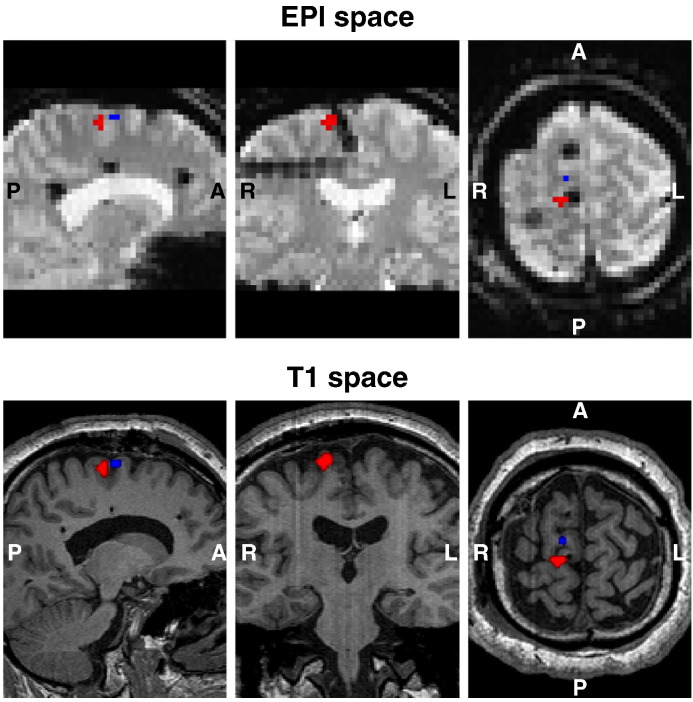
Representative example (patient ID: B, events of interest set: *S*_*2*_, see Table 1) of IED onset and width related BOLD changes (*p* < 0.05, uncorrected), within a 10 mm radius sphere centred at middle distance between the pair of icEEG contacts that shows the largest average IED (COI: PSMA 3–4). Positive BOLD changes (pBOLD) are showed in red, and negative BOLD changes (nBOLD) are showed in blue.
